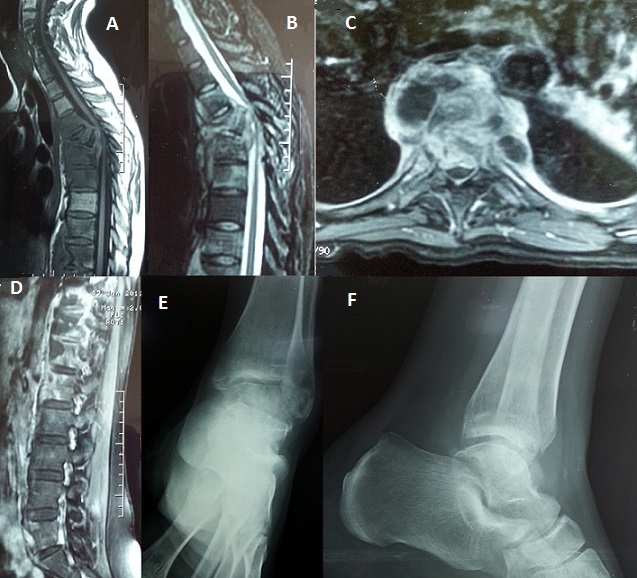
(A) IRM du rachis dorsal en coupe sagittale T1: destruction vertébrale de D4 avec recul du mur postérieur responsable d'une déformation dorsale avec compression médullaire; (B) IRM du rachis dorsal en coupe sagittale T2: destruction vertébrale de D4 avec recul du mur postérieur et compression médullaire, collection épidurale en regard de D3D4D5D6; (C) IRM médullaire en coupe transversale passant par D4: destruction vertébrale de D4 avec recul du mur postérieur; (D) IRM du rachis lombaire en coupe sagittale T2: atteinte de D10, D11, D12, L1 et L5 avec petite collection vertébrale; (E) radiographie de la cheville droite de face: absence de lyse osseuse; (F) radiographie de la cheville droite de profil: absence de signes en faveur d'une ostéo arthrite
